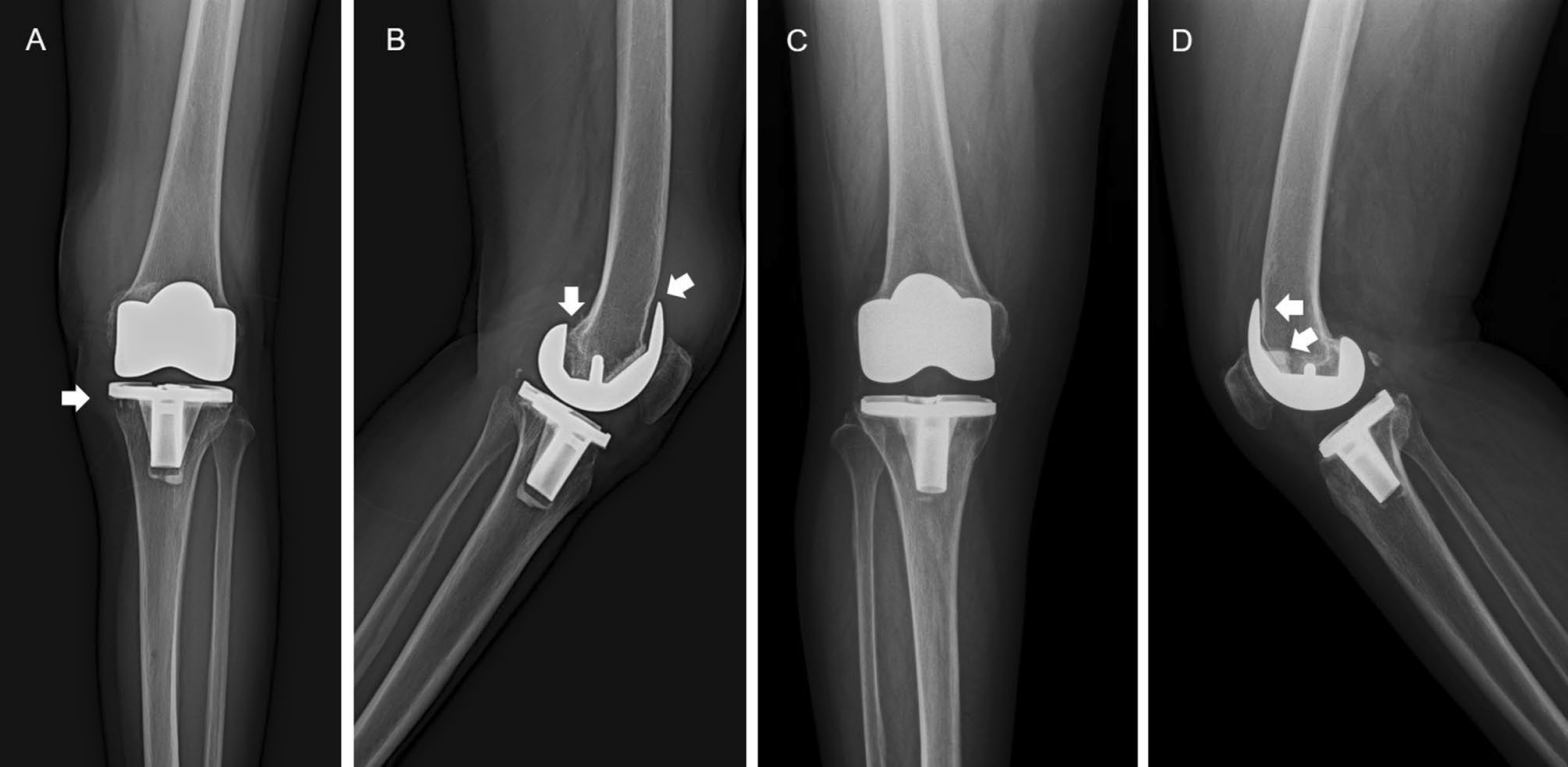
(A,B) Radiographs of Lt knee of a 70-year-old woman with taken 10 years after surgery revealing the NexGen high-flexion CR bearing prosthesis-fixed. (A) Anteroposterior radiograph. Radiolucent line is demonstrated zone 1 around tibial component according to the KSS system^7^. (B) Lateral radiograph. Radiolucent line is demonstrated zone 1, 4 around femoral component according to the KSS system. (C,D) Radiographs of Rt knee of a 72-year-old man with taken 10 years after surgery revealing the NexGen high-flexion LPS fixed-bearing prosthesis. (A) Anteroposterior radiograph. No radiolucent line is demonstrated around tibial component. (B) Lateral radiograph. Radiolucent line is demonstrated zone 1, 2 around femoral component according to the KSS system.
